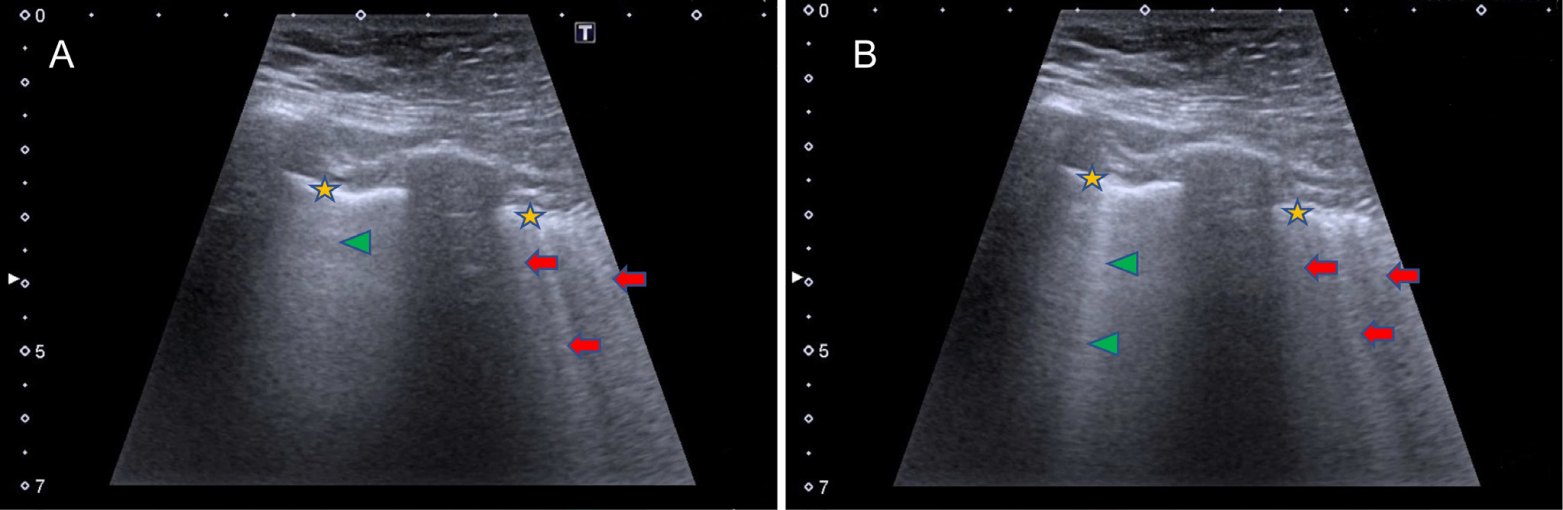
A-B. Lung ultrasound of right lateral upper lung field showing B-line pattern. Red arrows, B-Lines; Green arrowheads, coalescent B-Lines; Yellow stars, pleural line.
